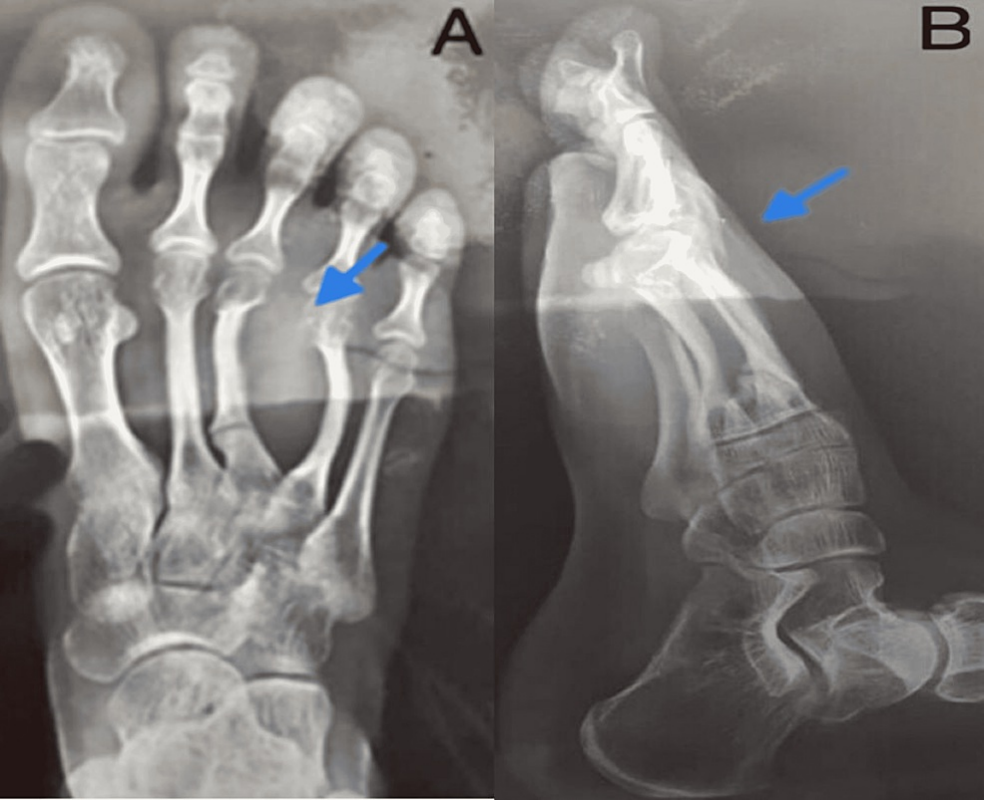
Plain right foot x-ray shows abnormal growth between the third and fourth digit in anteroposterior view (A) and lateral view (B).
